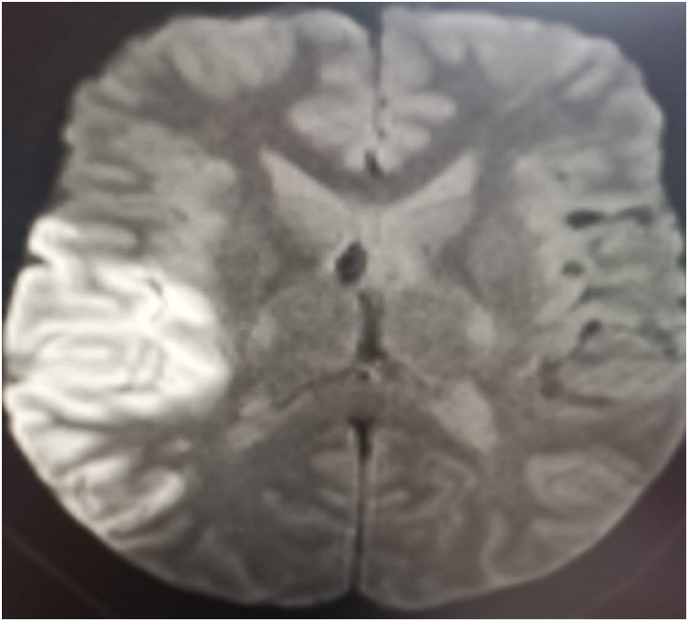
MRI brain with diffusion weighted images showed hyperintense lesion in the right parieto-occipital denoting acute infarction.
